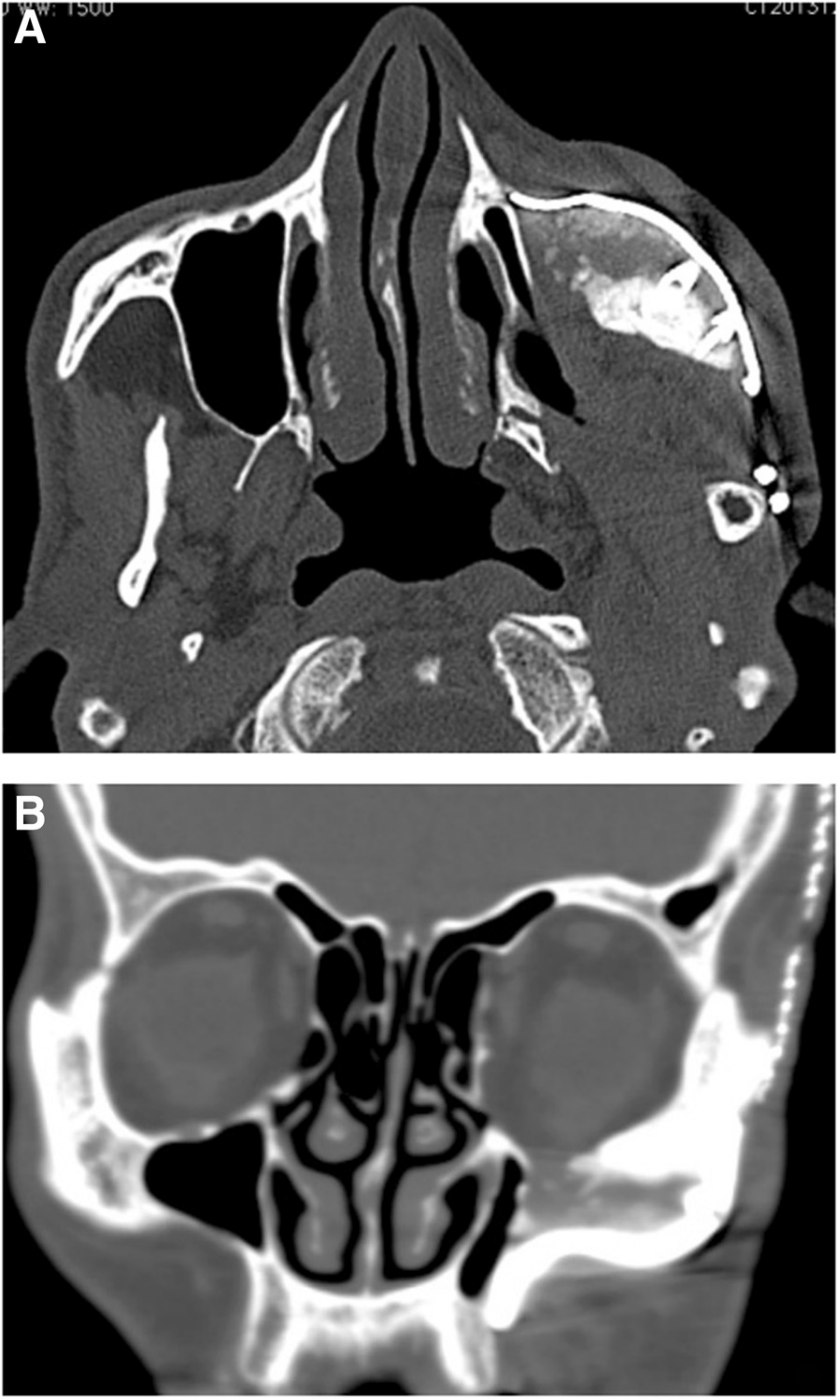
Postoperative axial CT scan showing the restoration of the symmetry and anterior projection of the zygomatic bone: (A) Axial, (B) Coronal.
